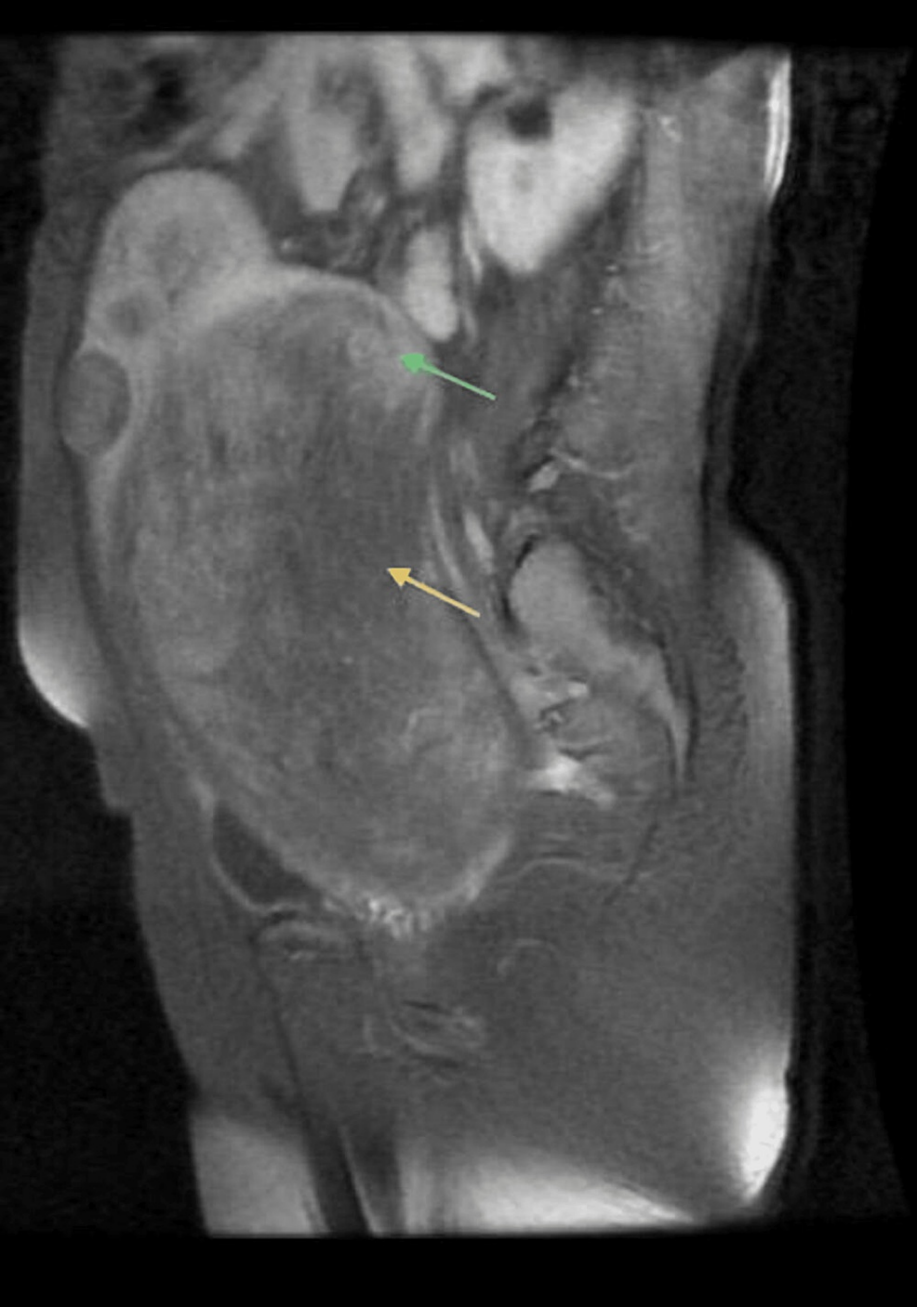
A T1-weighted MRI image with contrast shows an enhancing large mass (green arrow) and necrotic area (yellow arrow) in the uterine posterior wall.
